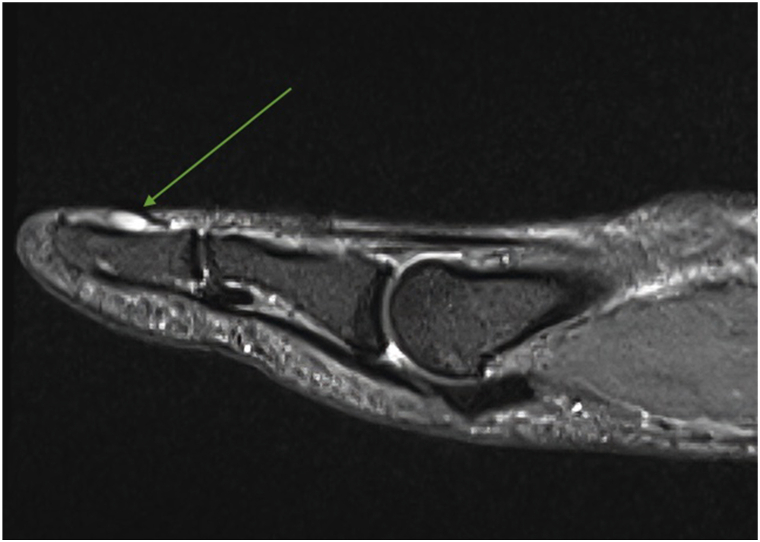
Magnetic resonance imaging of the right great toenail showcasing a glomus tumor as indicated by the *arrow*.
